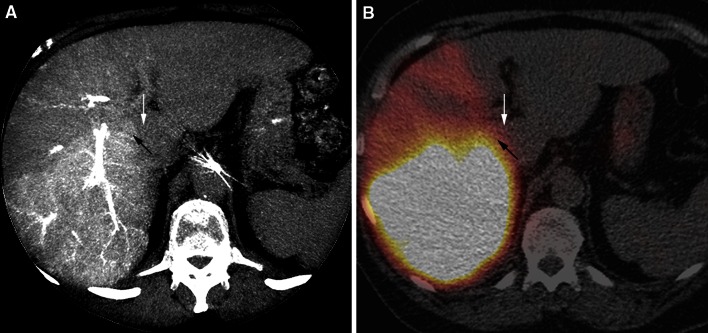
Comparison of C-arm CT A and SPECT/CT B in a patient with right lobar infusion of ^99m^Tc-MAA. The right part of segment 1 is perfused (*black arrow*), but the left part is non-perfused (*white arrow*). Note the free pertechnetate in the stomach on SPECT/CT (B)
